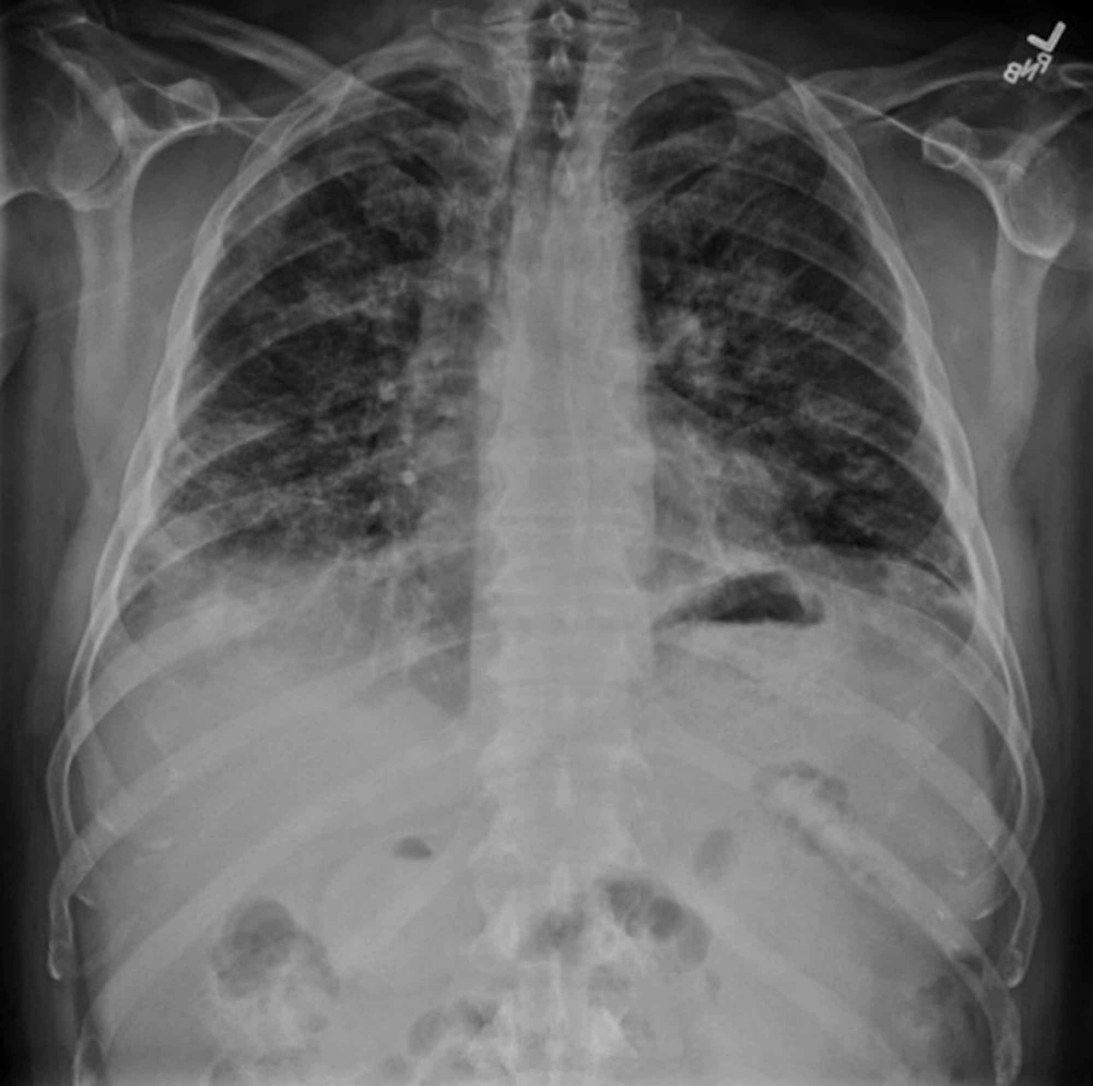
Chest X-ray demonstrating bilateral patchy interstitial infiltrates
